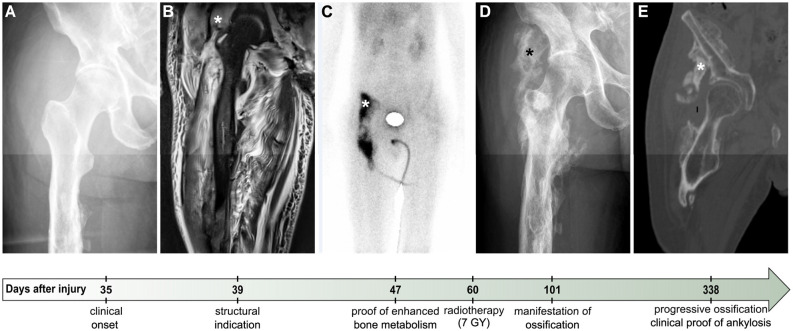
Exemplary clinical course of severe Heterotopic Ossification (ID 13). Plain X-ray (A,D), MRI (B), scintigraphy (C), and CT (E), in chronological sequence of the clinical course from the onset of symptoms to the decision on surgical resection of HO. “Days after injury” denote the time elapsed since SCI. Depicted stars (*) point to areas of ossification. Four days after first clinical indications of potential HO medical diagnostics were initiated (day 39 after SCI), with plain X-ray still lacking reliable proof of ossification (A). On the same day, MRI showed suspicious but rather nonspecific diffuse T2 hyperintense muscle signal behavior in the vicinity of the femoral head (B). Forty-seven days after SCI, enhanced bone metabolism detected by scintigraphy confirmed the previously suspected diagnosis of HO (C). Despite a performed single-time radiotherapy with 7 Gy on day 60, a clinically relevant ossification was proven by CT more than 2 months after the onset of symptoms (D). Ankylosis occurred roughly one year after SCI (E) and led to a surgical resection of the ossification (day 408 after SCI), immediately after a second single radiotherapy with 7 Gy the day before.
